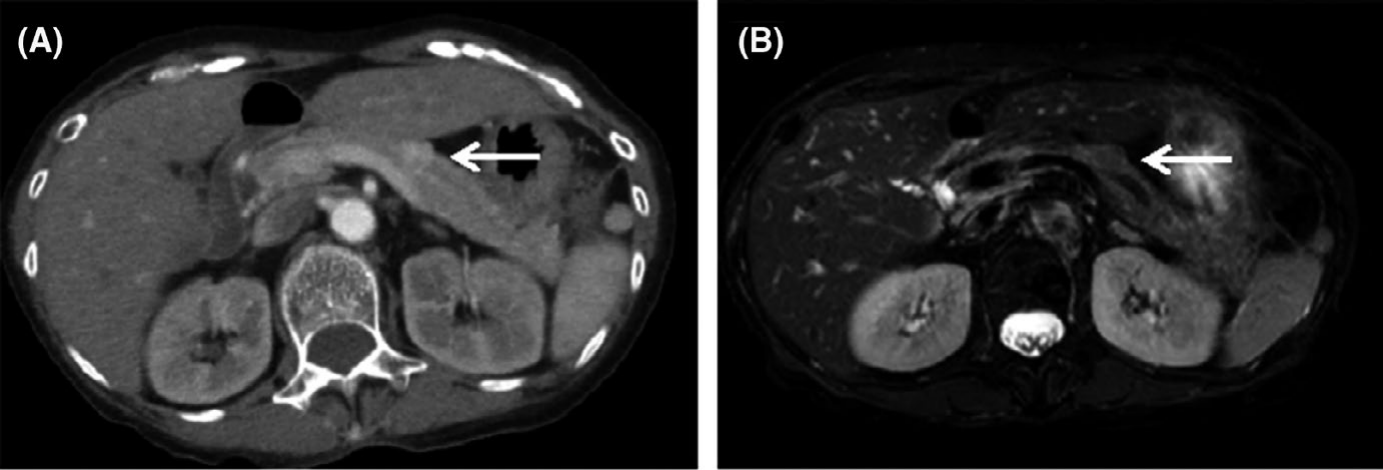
Computed tomography (CT) and magnetic resonance imaging (MRI) findings. CT (A) shows a 9‐mm‐diameter nodule (arrow) in the pancreatic body, and MRI (B) shows a low‐signal area at the same site (arrow)
